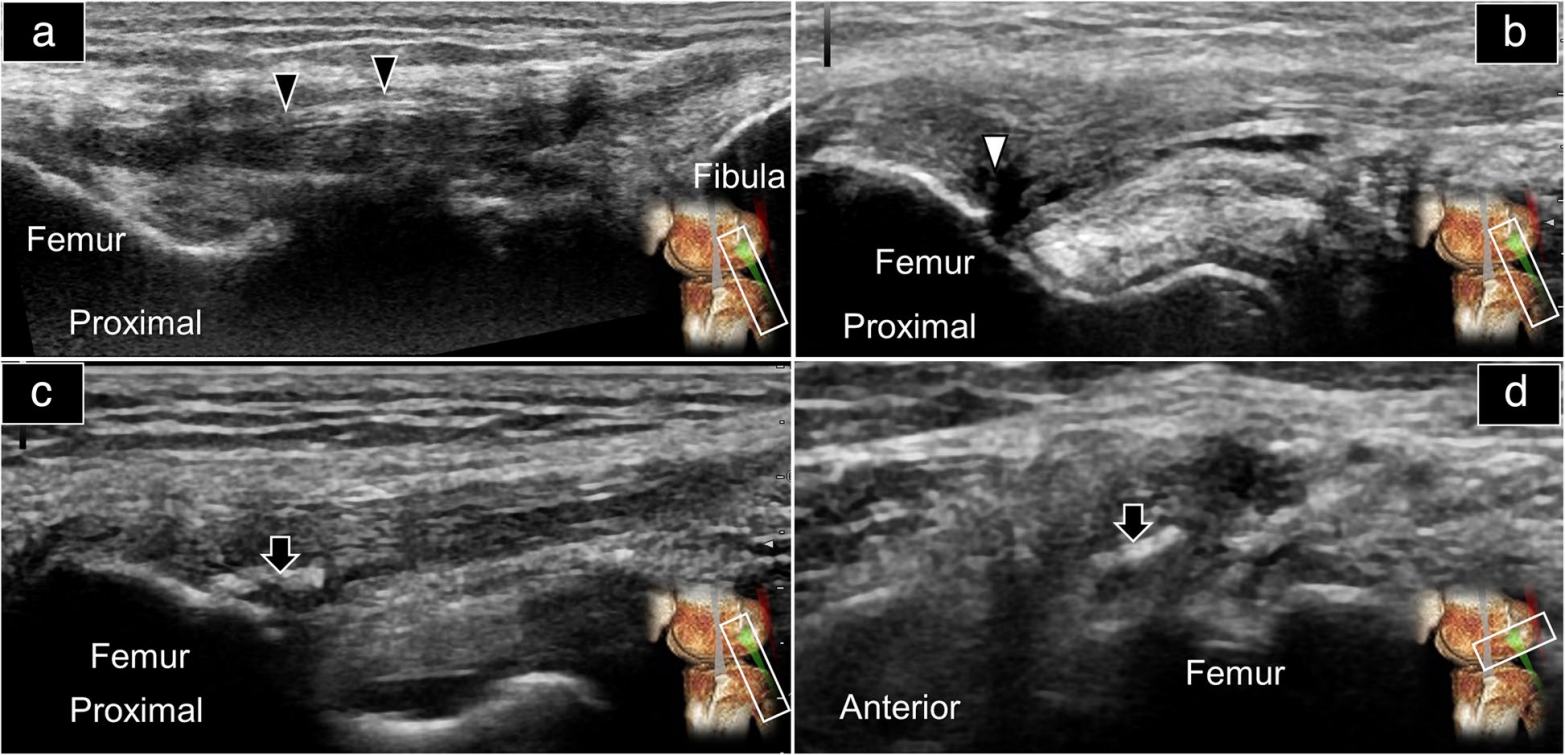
Ultrasound imaging using the long-axis approach revealed the thickened and hypoechoic changes (black arrowheads) (a), an anechoic region consistent with a partial thickness tear (white arrow) (b), and calcification (black arrows) in long-axis (c) and short-axis (d) views of the lateral collateral ligament. Please also reference Fig. 5A for the normal appearance of the lateral collateral ligament
